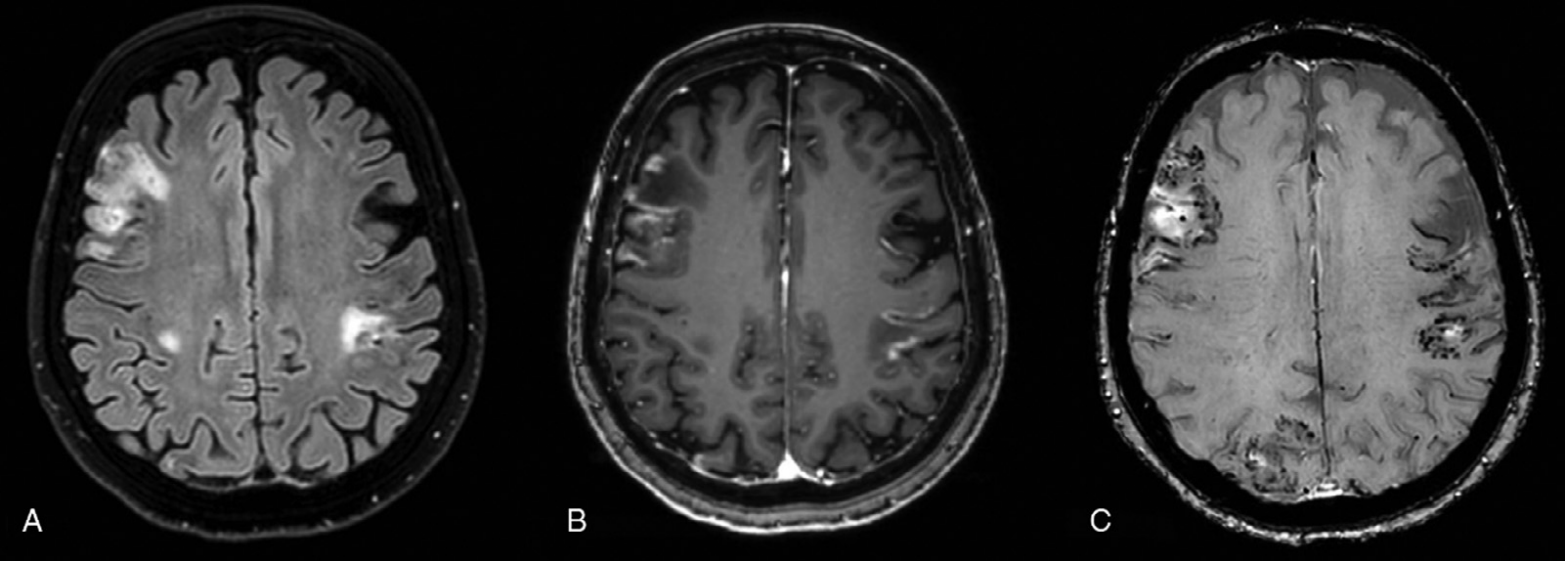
CASE 1 (A) FLAIR sequence showed abnormal cortical-subcortical lobar hyperintensities in both cerebral hemispheres. (B) Postcontrast axial T1 images demonstrated marked gyral enhancement in the same areas. (C) Multiple and bilateral cortical–subcortical microhemorrhages were seen on postcontrast Susceptibility weighted images corresponding to FLAIR cortical-subcortical hyperintensities.
